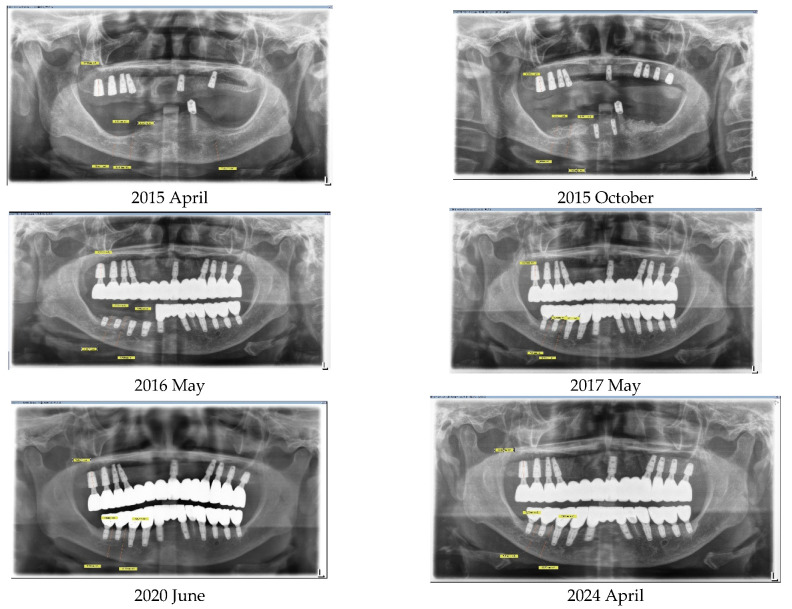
Panoramic X-ray images displaying the perioperative state along with the annual changes postoperatively.
